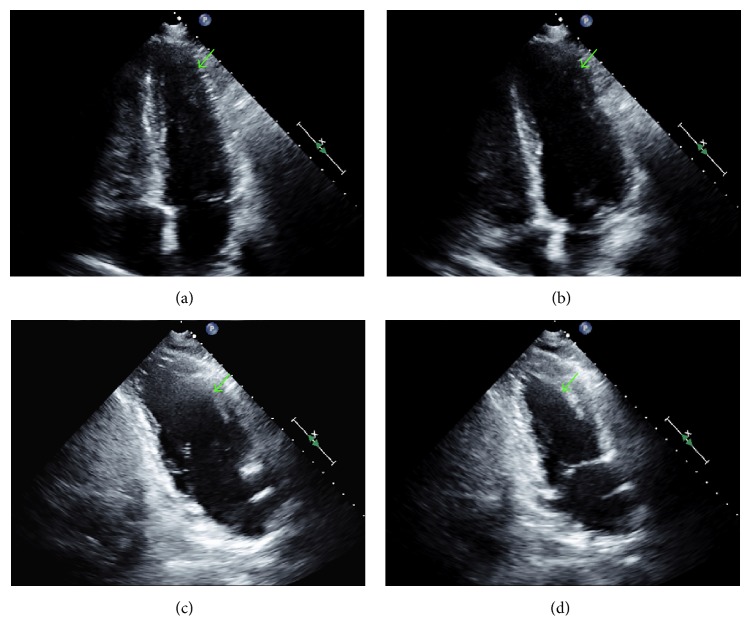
Repeat transthoracic echocardiogram confirming complete recovery of ventricular systolic function and resolution of the acute wall motion abnormalities, supporting the diagnosis of stress cardiomyopathy. Systolic (a) and diastolic (b) apical 4-chamber views and systolic (c) and diastolic (d) apical 2-chamber views reveal normal motion of the ventricular apex (arrows).
